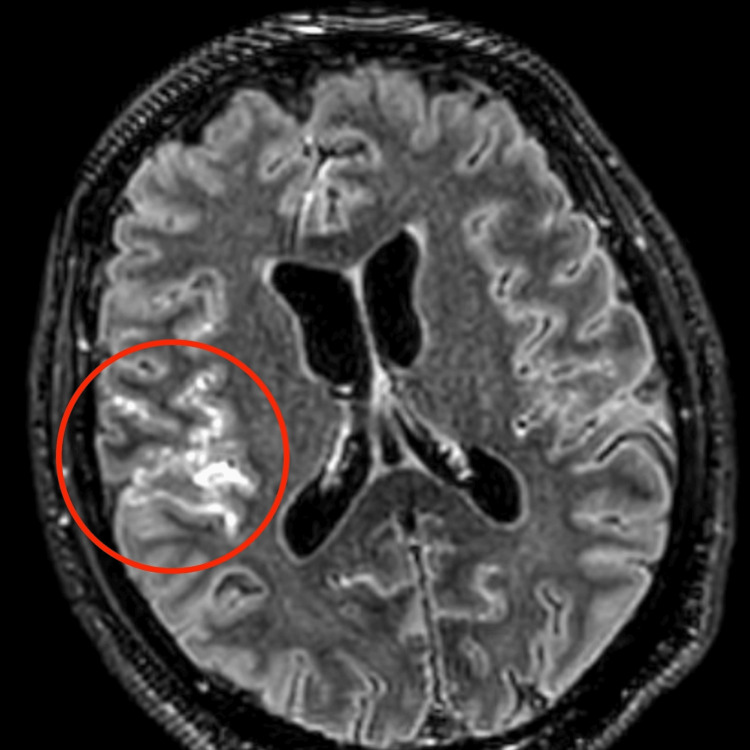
Axial post-contrast FLAIR image demonstrating focal leptomeningeal enhancement in the right posterior frontal region, adjacent to the insular cortex, consistent with leptomeningitis in the setting of tuberculous meningitis (red circle indicates the area of leptomeningeal enhancement). FLAIR: fluid-attenuated inversion recovery
